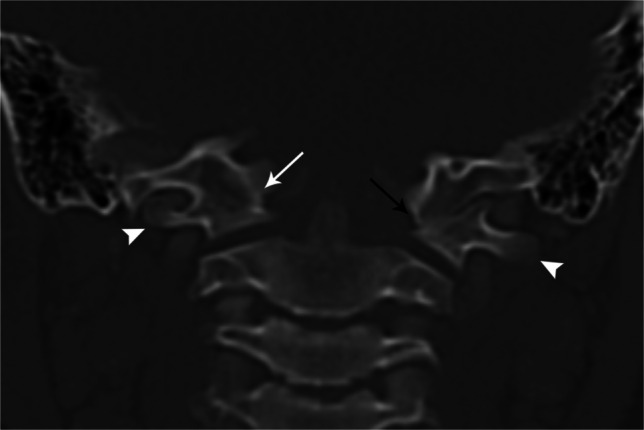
Coronal CT of the neck in a 14-year-old male demonstrates complete atlanto-occipital assimilation on the right (*white arrow*) and incomplete atlanto-occipital assimilation on the left (*black arrow*). The presence of the transverse processes of C1 (*arrowheads*) helps distinguish assimilation from C1 aplasia
